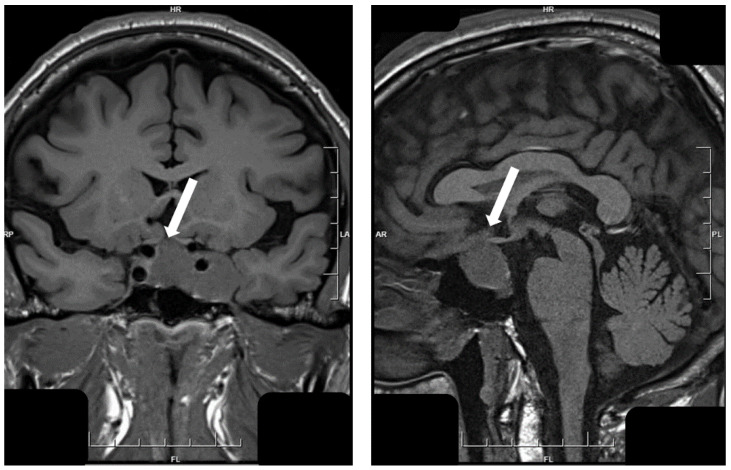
Magnetic resonance imaging of hypothalamic gangliocytoma with sparsely granulated somatotroph tumor. This tumor fills the sella turcica and the left cavernous sinus but also shows a thin attachment to the hypothalamus (white arrows).
